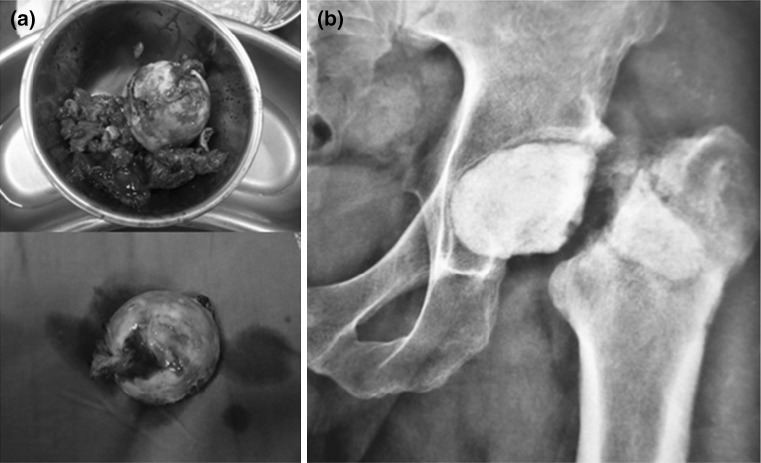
In the first stage a radical debridement of the joint removing the hardware plus infected femoral head and soft tissue b post-operative X-ray with the spacer in place
